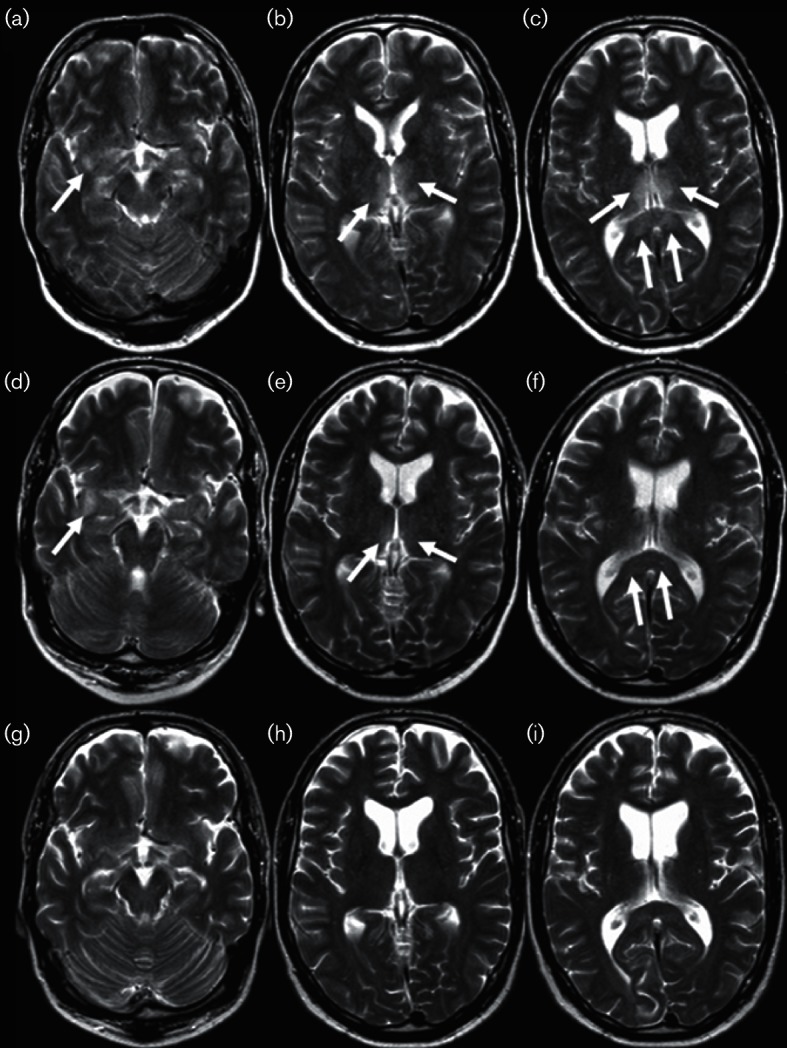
Brain MRI of the patient in our case report. Axial T2-weighted magnetic resonance images at the time of the initial presentation (a–c), 1 week later (d–f) and 2 months later (g–i) demonstrating the distribution and evolution of lesions. On the initial MRI, focal T2-hyperintense lesions were identified in the subcortical white matter of the insula (a, arrow), bilateral in the thalamus (b, arrows; c, top two arrows) and the splenium of the corpus callosum (c, bottom two arrows). One week later the lesions regressed in size. Only the lesion in the insula showed mild progression (d, arrow). On the last follow-up scan 2 months later (g–i), none of the focal lesions were visible anymore. In addition, no neurodegenerative sequelae could be identified.
